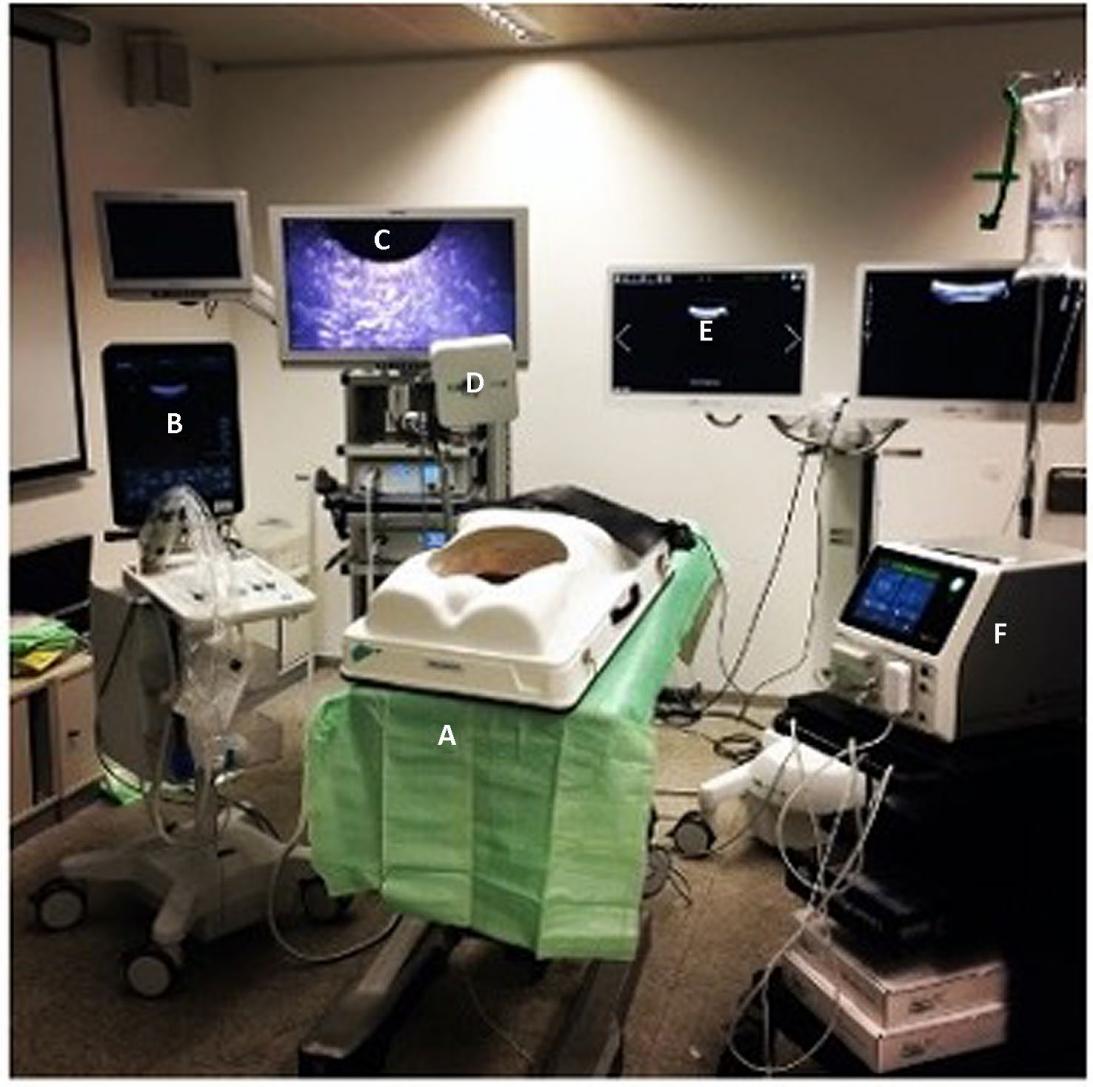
Experimental setup (A laparoscopic torso trainer, B Ultrasound device, C laparoscopic tower, D electromagnetic field generator, E CAS-One System, F Ablation power unit)
